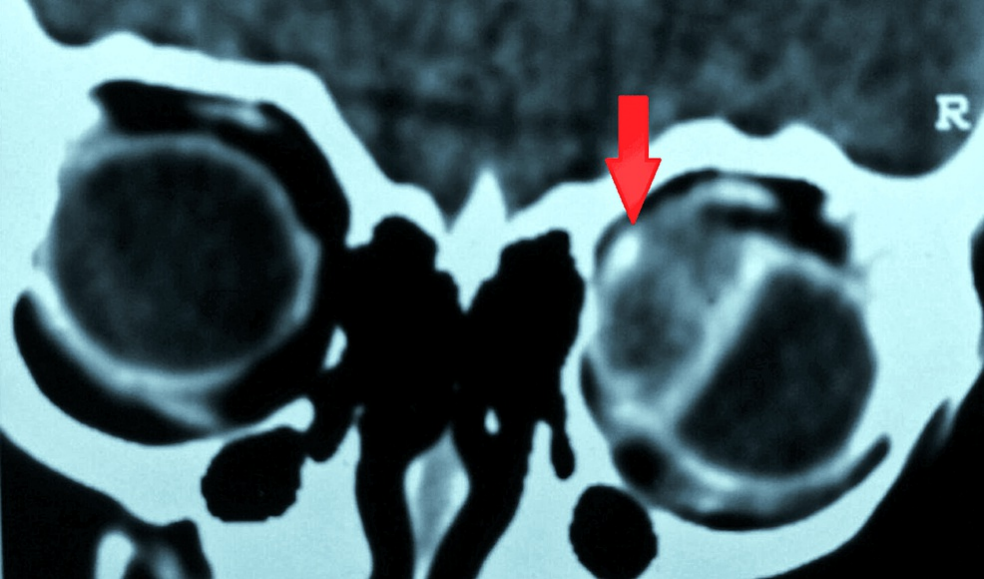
CT scan of orbit CT: computed tomography Image credit: Dr. Nivesh Gupta
